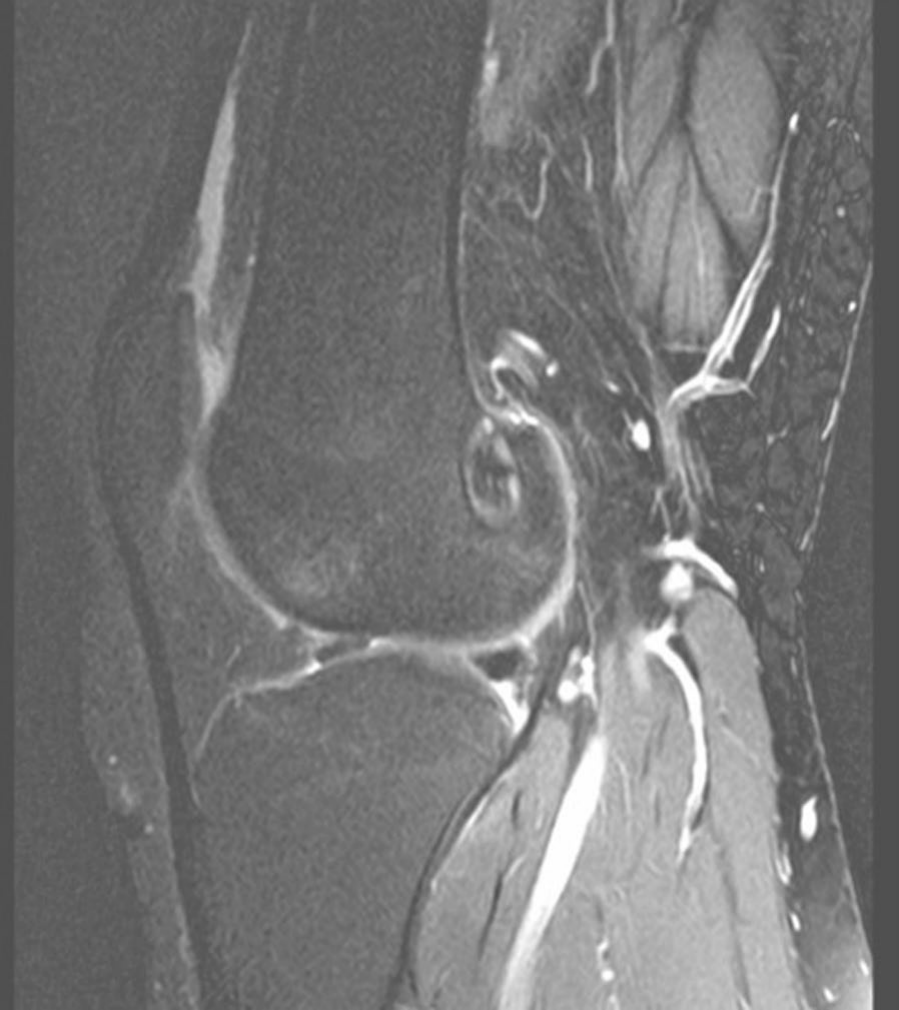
Sagittal magnetic resonance imaging of the fibrous interzone in the femoral tunnel in STGPI graft
